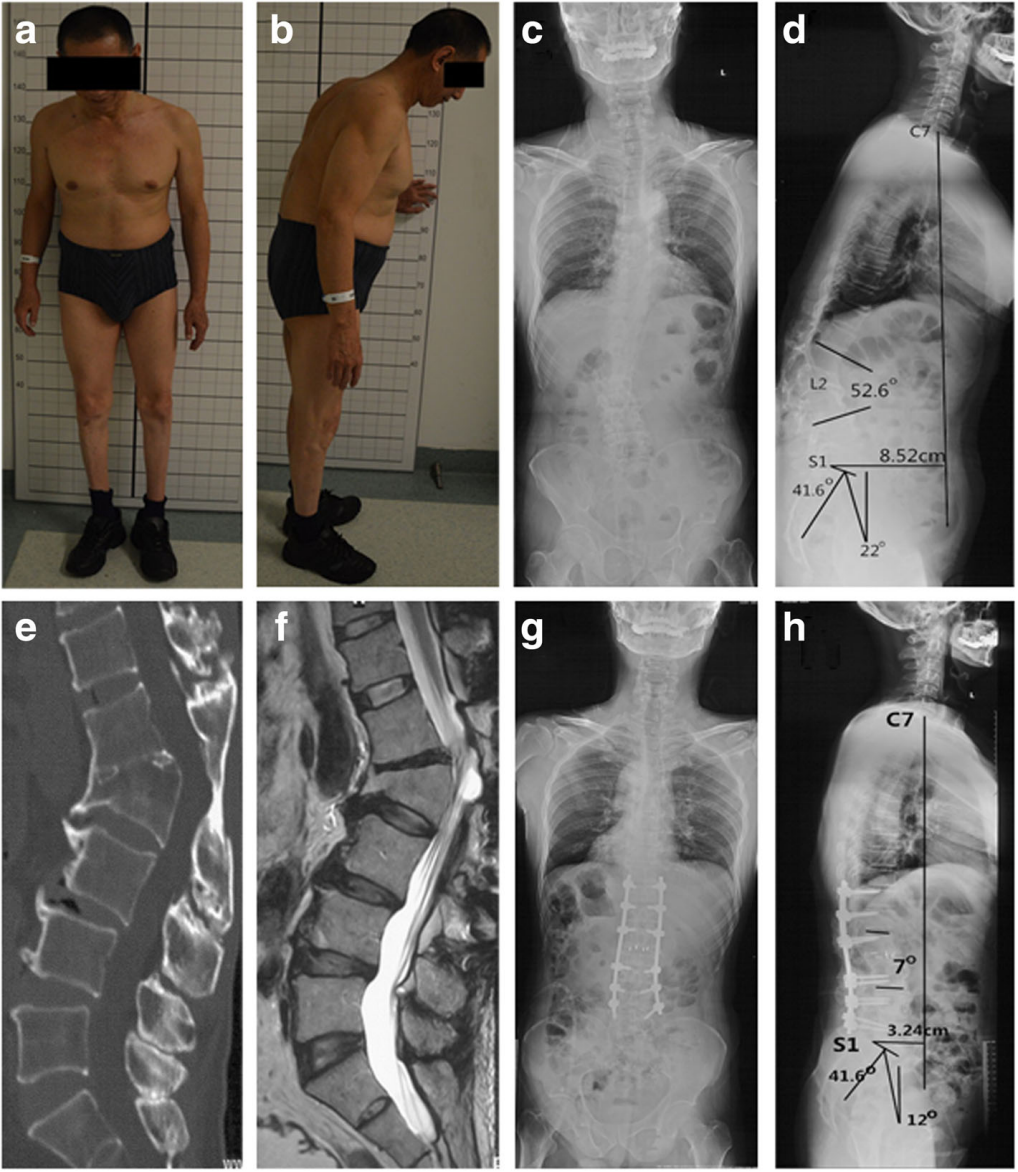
A 55-year-old male patient (a, b) who is suffering from severe back pain and numbness in the right thigh for more than 12 months due failure of conservative treatment. Preoperative anteroposterior (c), lateral radiographs (d), and CT (e), MRI (f) show that the apex of kyphosis is located at L2 with PI 41.6°, PT 22°, SS 20°, SVA 8.52 cm, and the Cobb angle is 52.6°. Three years postoperatively anteroposterior (g) and lateral radiographs (h) of L2 with a 7 Cobb angle, PT 12°, SS 37°, SVA 3.24 cm, and bony fusion was achieved
